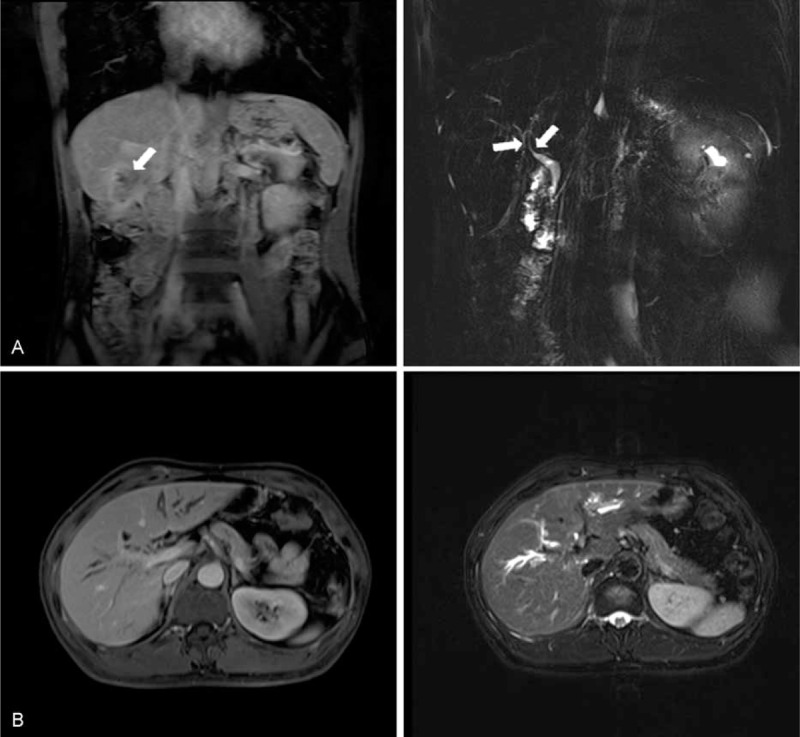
Preoperative magnetic resonance cholangiopancreatography images. (A) Coronal reconstructed image revealed an irregular and abnormal signal intensity lesion (approximately 3.7 cm) in segment VI of liver mimicking malignancy (left), and the obstructed bile duct (right). Arrows show the stricture of the intrahepatic bile ducts caused by the cholangiocarcinoma. (B) Transversal sections on T1-weighted (left) and T2-weighted (right) images showed a slight enhancement of the common bile duct. Dilation and irregularity of biliary tracts were also obvious.
